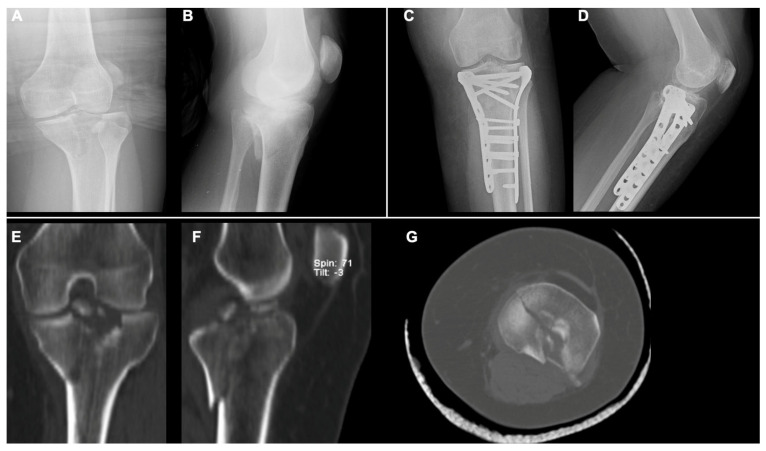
Radiographic images of a bi-condylar (Schatzker V) tibial plateau fracture in a 50-year-old male patient: AP and LL views of X-rays at preoperative period (A,B) and at 41-month follow-up (C,D), respectively. Preoperative CT scan images in coronal (E), sagittal (F), and (G) axial views, respectively.
